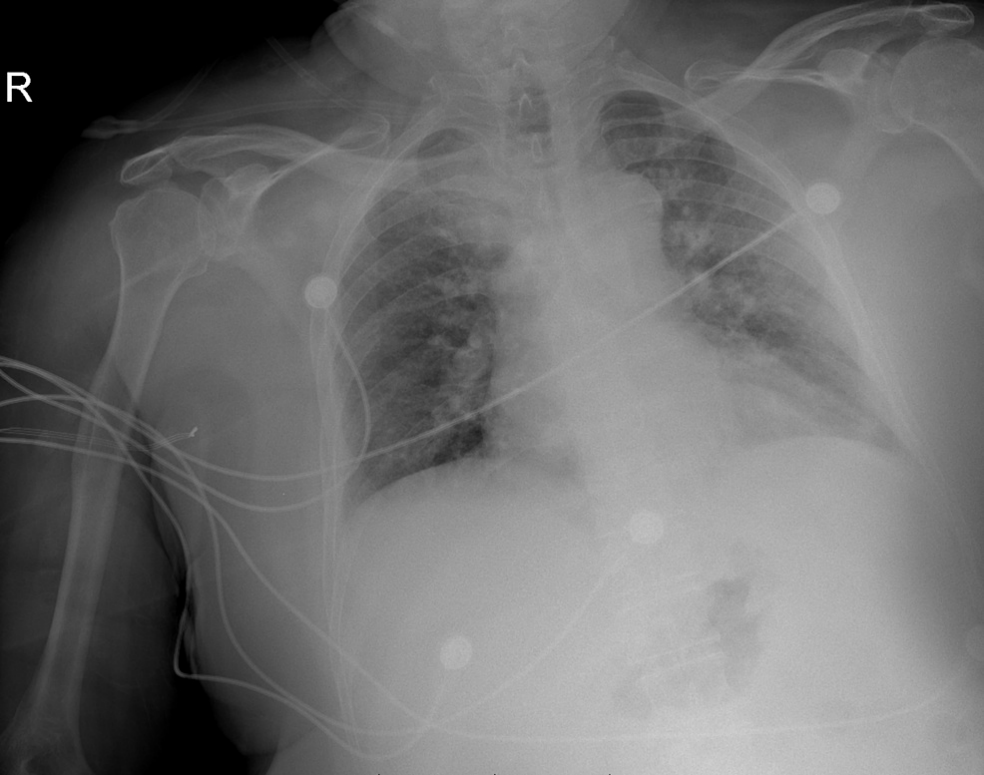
Chest X-ray showed bilateral lung infiltration
